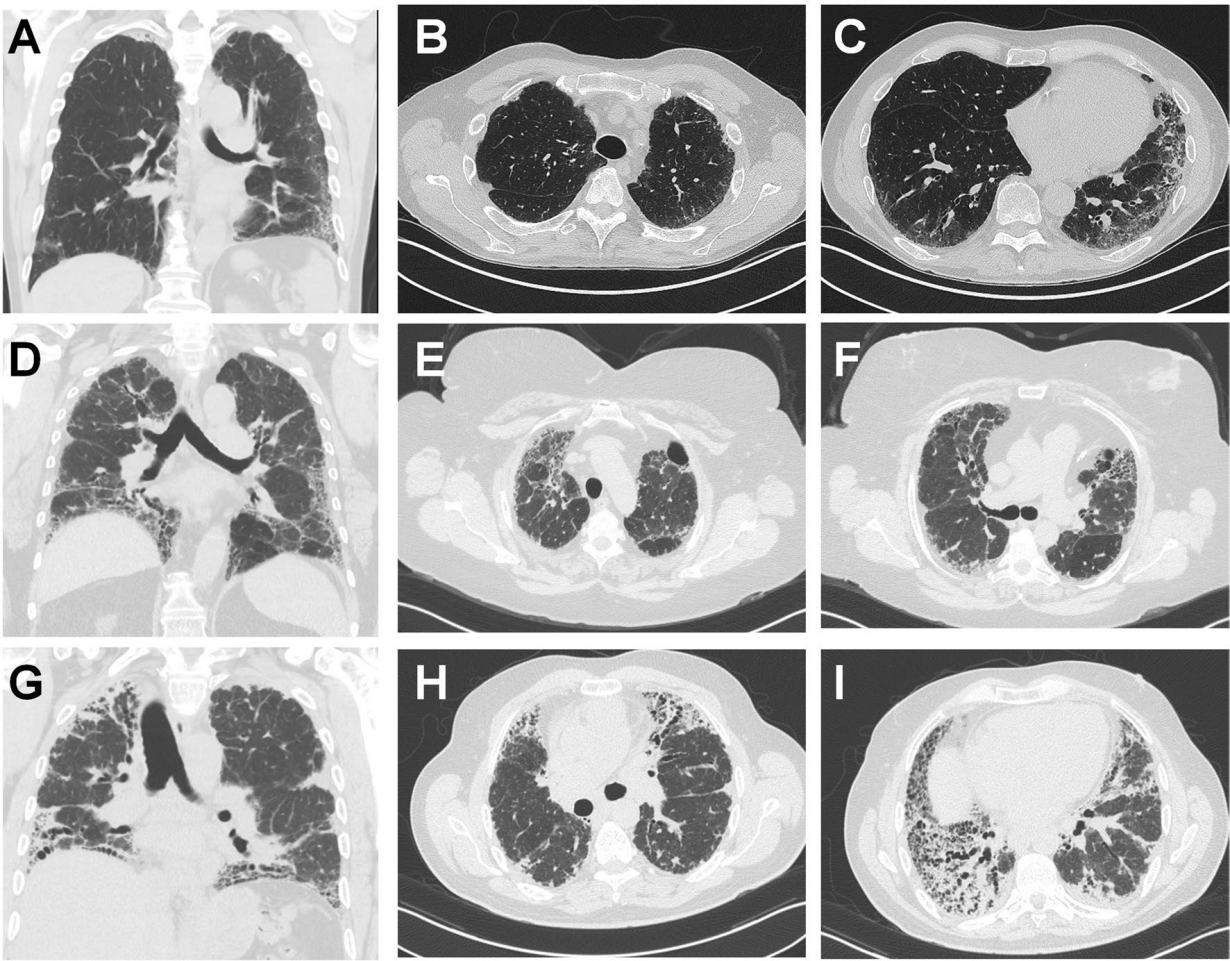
Representative high-resolution computed tomography (HRCT) coronal (A, D, and G) and axial (B, C, E, F, H, and I) images from adults with pulmonary fibrosis in the setting of pathogenic variants in telomere-related genes. A–C A 76-year-old male heterozygous for RTEL1 (c.3724 + 139 G > A). D–F A 64-year-old female heterozygous for *TERT (*c.1864 C > T, (p.R622C)). G–I A 59-year-old male heterozygous for TERT (c.2030 G > A, p.Gly677Asp)
